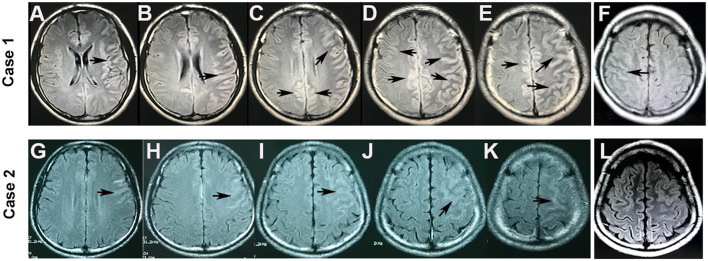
Brain MRI of cortical lesions in cases 1 and 2. FLAIR hyperintensity was seen in the bilateral cerebral cortex in case 1 (A–E) and in the left hemispheric cortical region in case 2 (G–K) (arrowheads). However, the FLAIR hyperintensities in the cortical regions became shallow and fewer after 3 months in case 1 (F) and disappeared after 1 month in case 2 (L).
